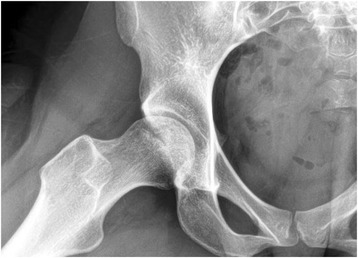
This AP x-ray was done 2 weeks post decompression. The patient was asymptomatic at this point, and x-ray shows a normal femoral head
